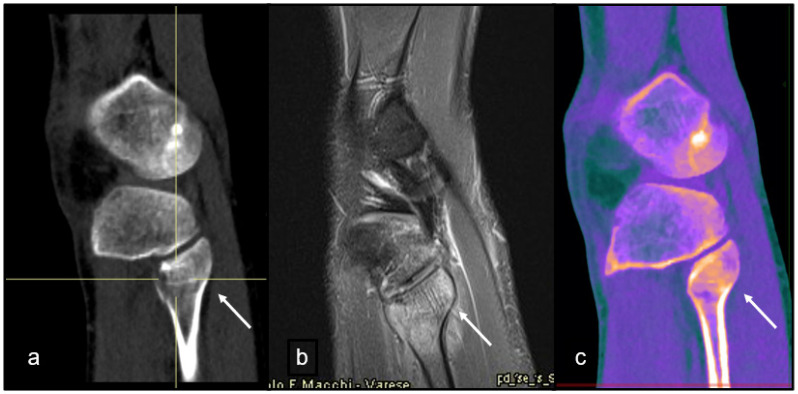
A 25-year-old male, sprain injury. (a) Coronal CT image, baseline acquisition of the knee shows no cortical alterations. (b) Sagittal MRI acquisition in PD/T2 FAT-SAT demonstrates edema of the fibular head, indicating acute post-traumatic interstitial hemorrhage (white arrow). (c) Sagittal DL-SCT image, post-processed with the IntelliSpace software, highlights the presence of edema through increased electron density, visualized in orange coloring (white arrow). Color bar legend. White: normal cortical bone; orange: intermediate to high ED; purple: low ED; blue: very low ED, consistent with normal bone marrow composition rich in fat.
